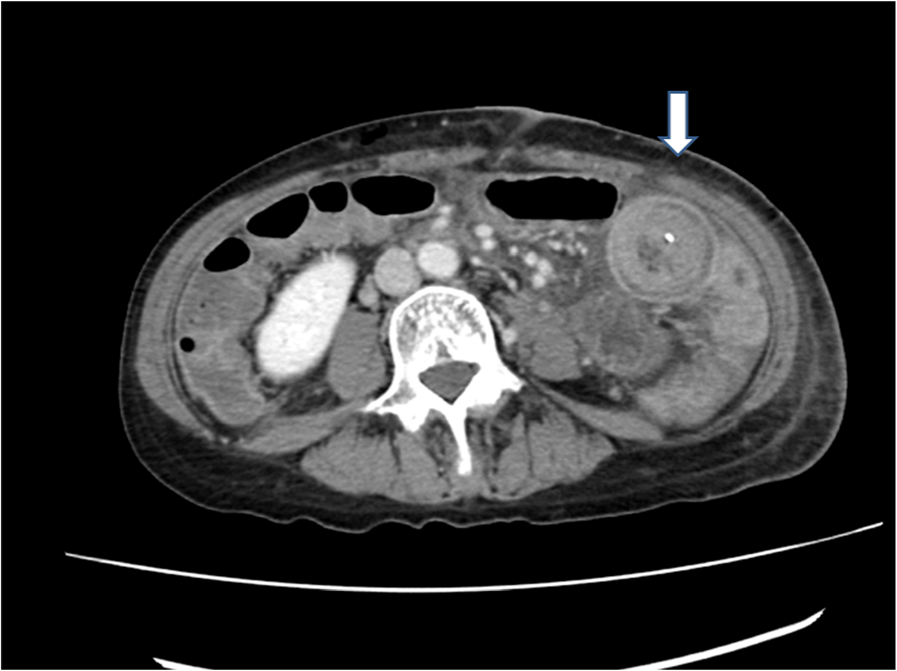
Abdominal computed tomography. A multiplex ring-formed mass connected with the small intestine.
